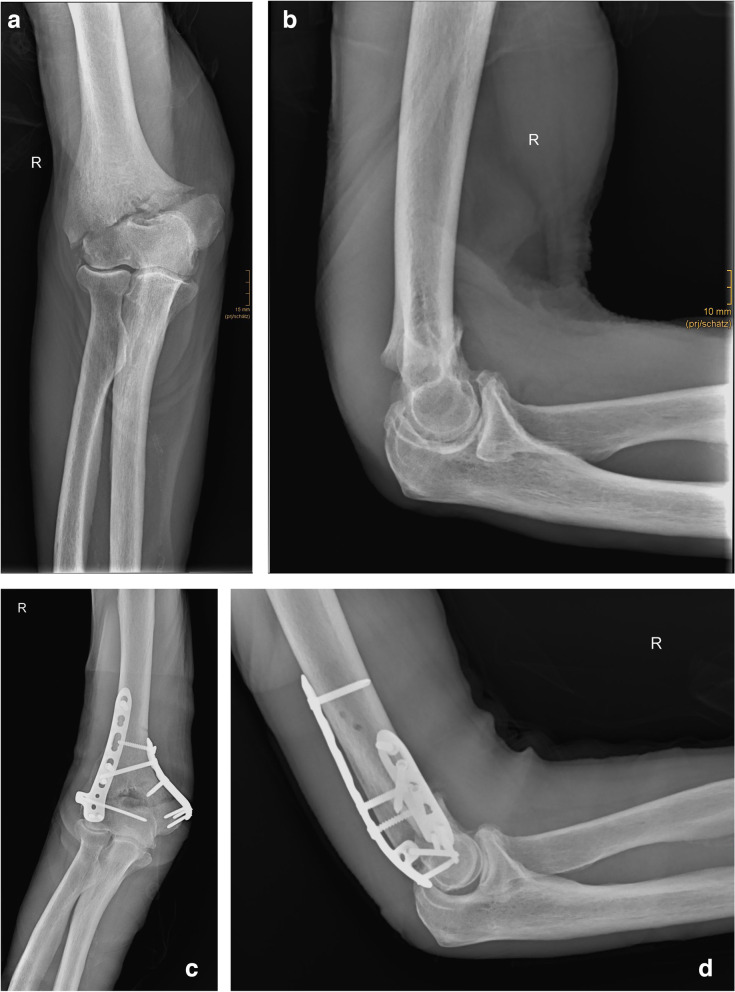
Extraarticular fracture of the distal humerus (AO 13 A3) in a patient. a,b Preoperative X-ray shows distal humerus fracture AO 13 A3. c,d Postoperative X-ray control
